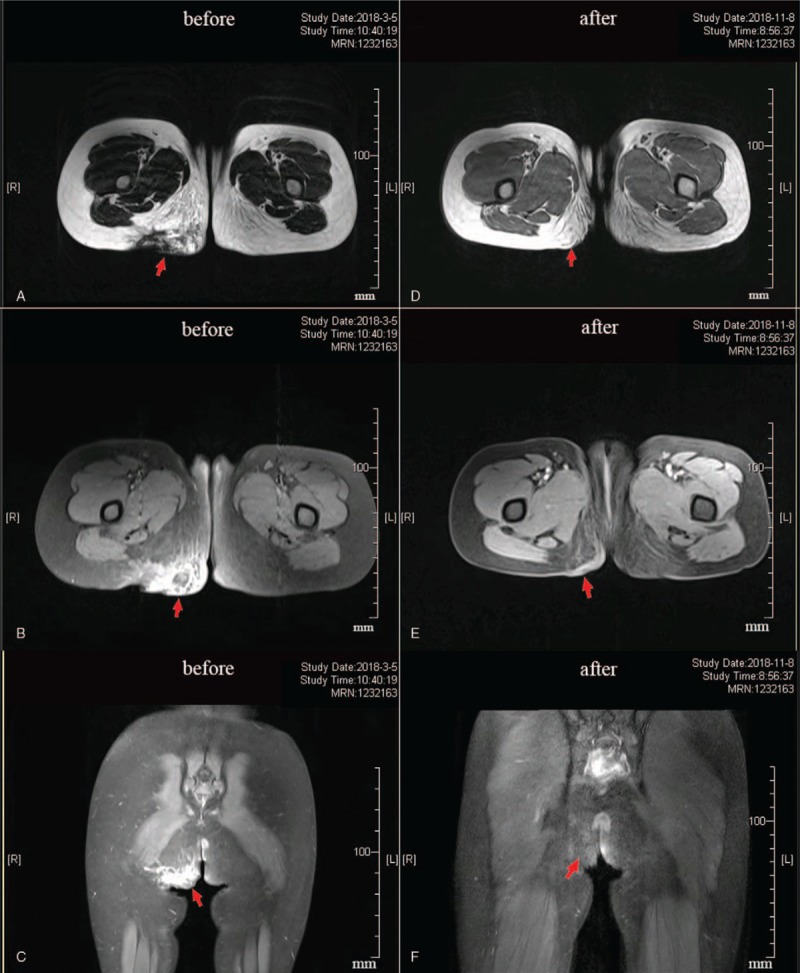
Doppler ultrasonography after treatment: Subcutaneous strip-like anechoic dark area (arrow), with clear boundary, about 29 mm in length, no blood flow signal was detected by CDFI (color Doppler flow imaging). Combined with the medical history, local subcutaneous effusion after hemangioma treatment was considered. (A) Slitting, (B) crosscut.
